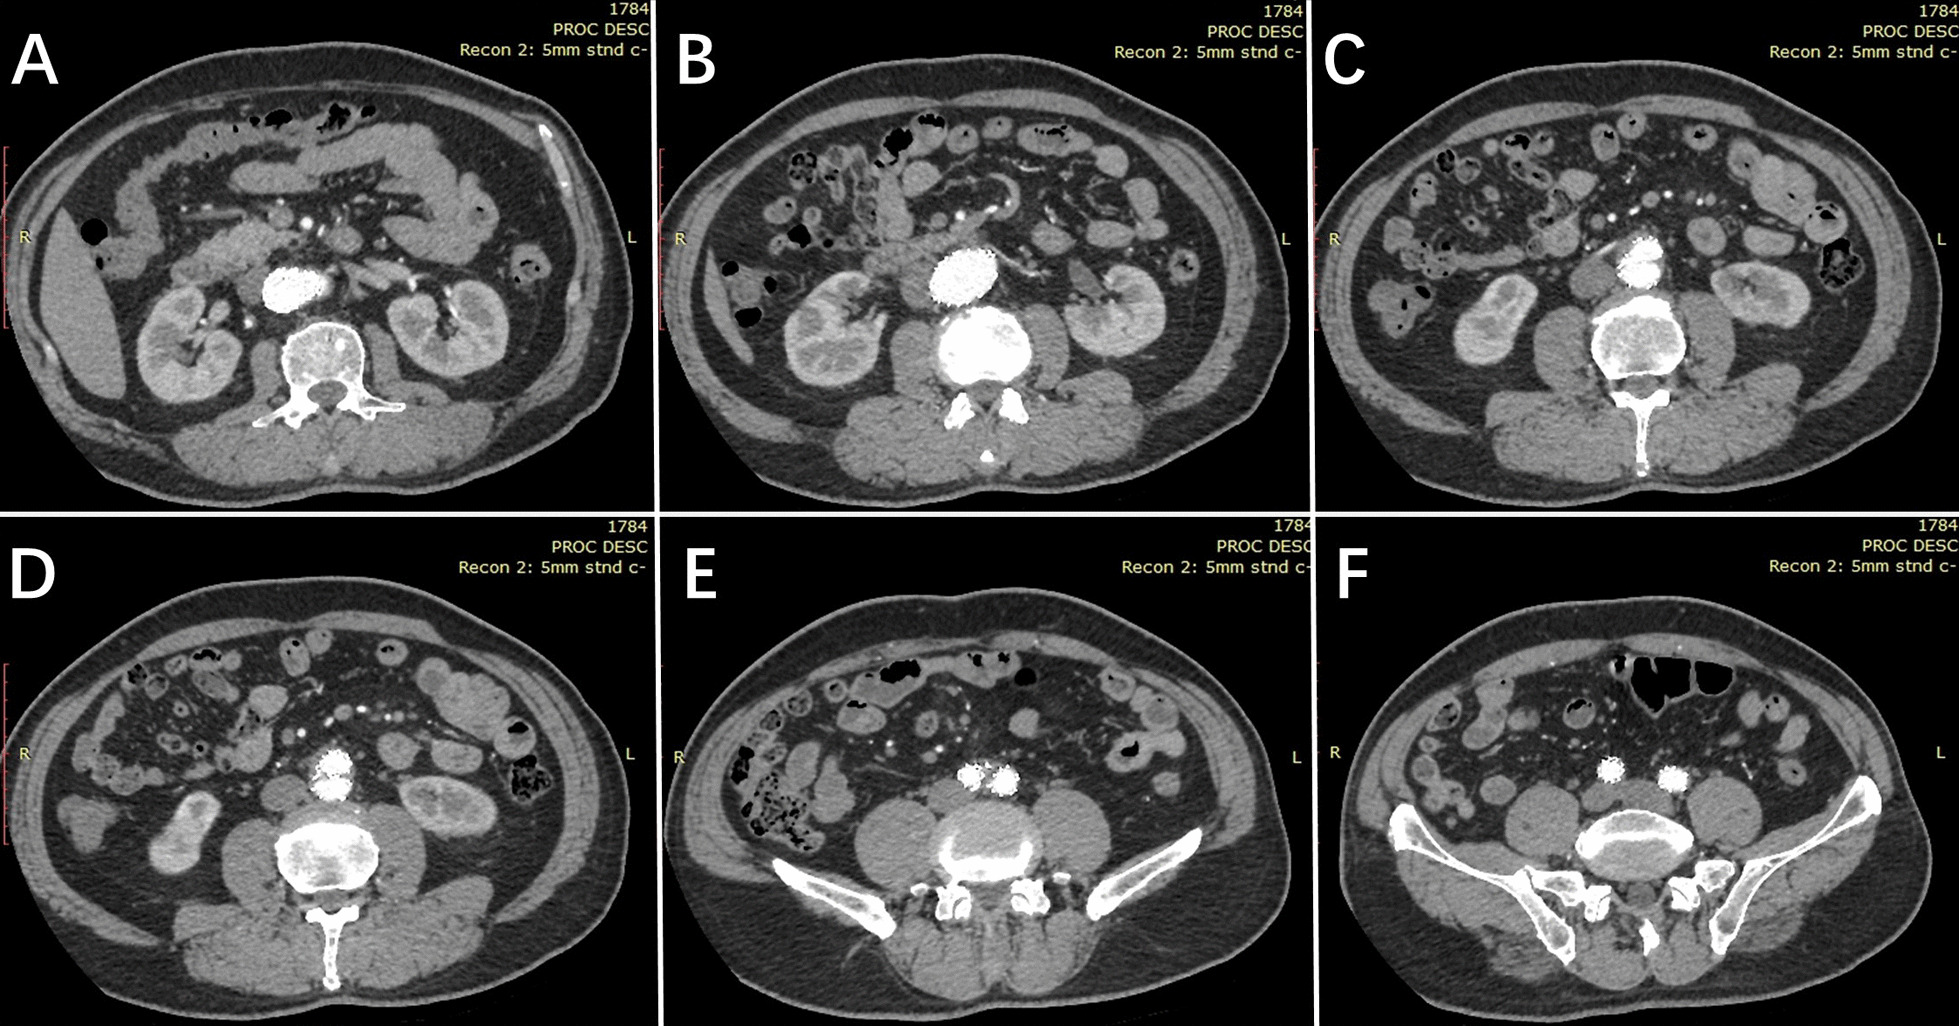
Postoperative axial-view surveillance computed tomography scans. A Abdominal aortic stent graft at the proximal end. B Normal perfusion of the left renal artery. C Normal perfusion of the right renal artery. D Bilateral common iliac artery stent grafts at the proximal end. E Normal perfusion in the bilateral common iliac artery stent grafts. F Bilateral common iliac artery stent graft at the distal end
